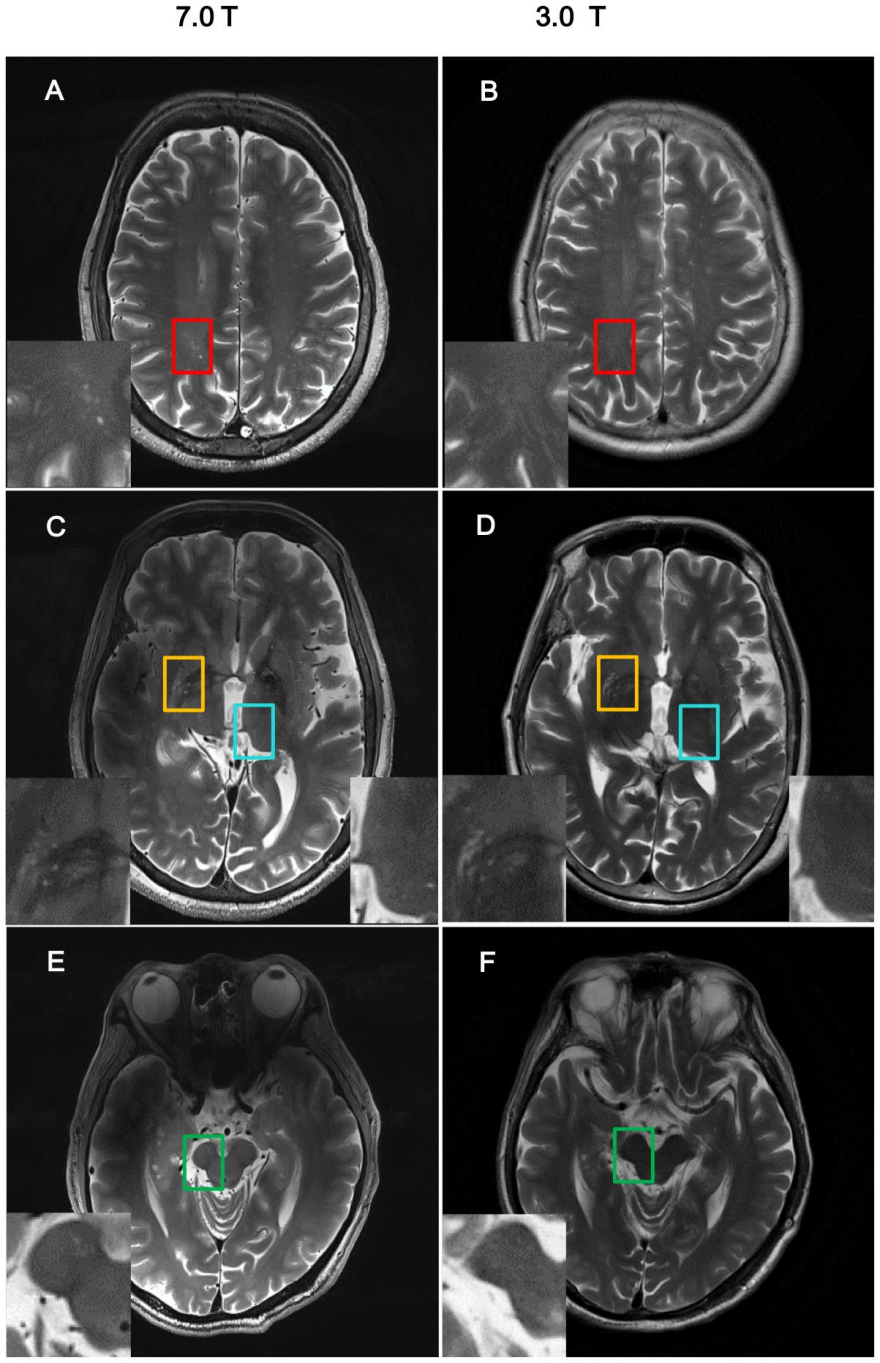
Comparison of imaging resolution between 7T and 3T MRI for nPVS. Example of comparisons of resolution of nPVSs on T2 weighted images acquired by 7.0T MRI vs 3.0T MRI on the same study participant. (A, B) Indicate centrum semiovale with red square; (C, D) indicate yellow squares for basal ganglia and blue squares for thalamus; (E, F) is midbrain with green squares. nPVS, normal-sized perivascular space.
